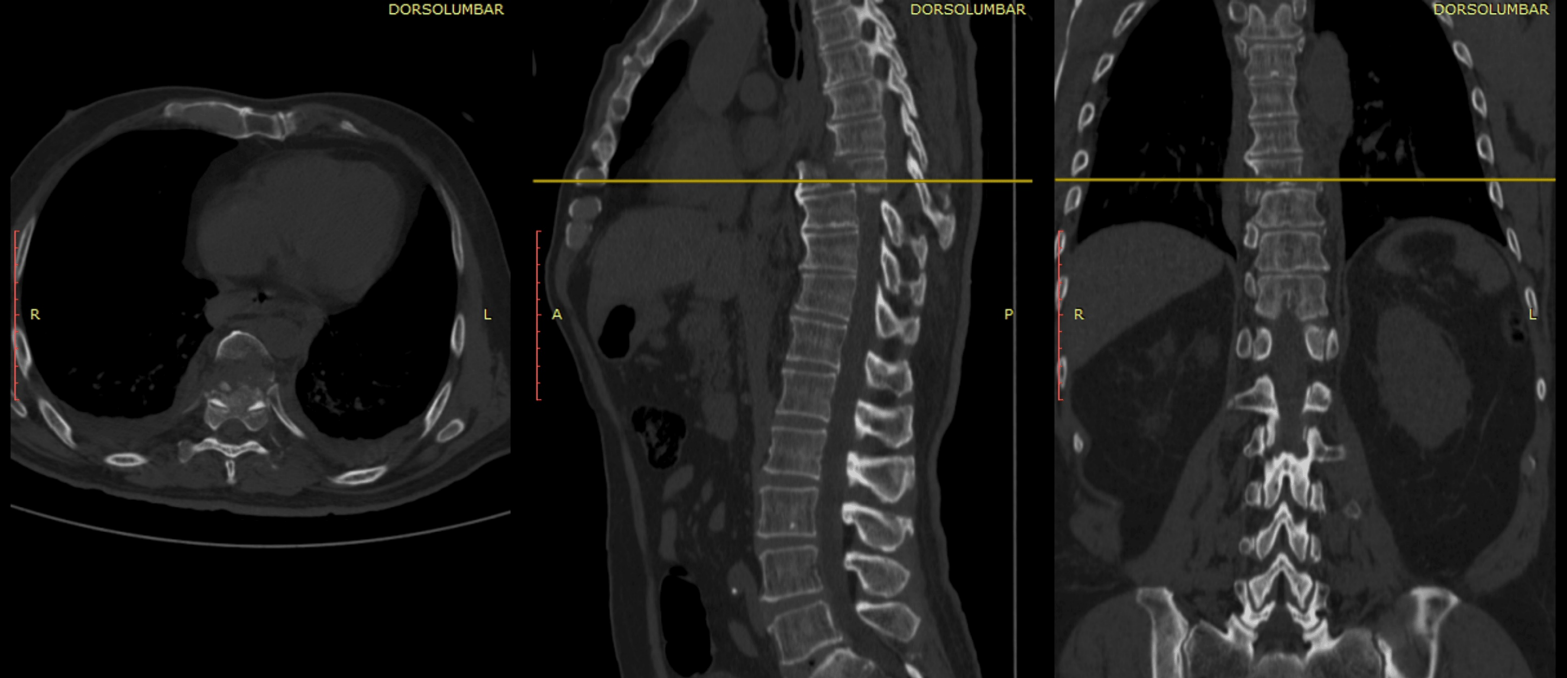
Case 3 CT scan of the initial injury and the displacement of the T8 body over T9 are visible in axial, coronal, and sagittal views.
